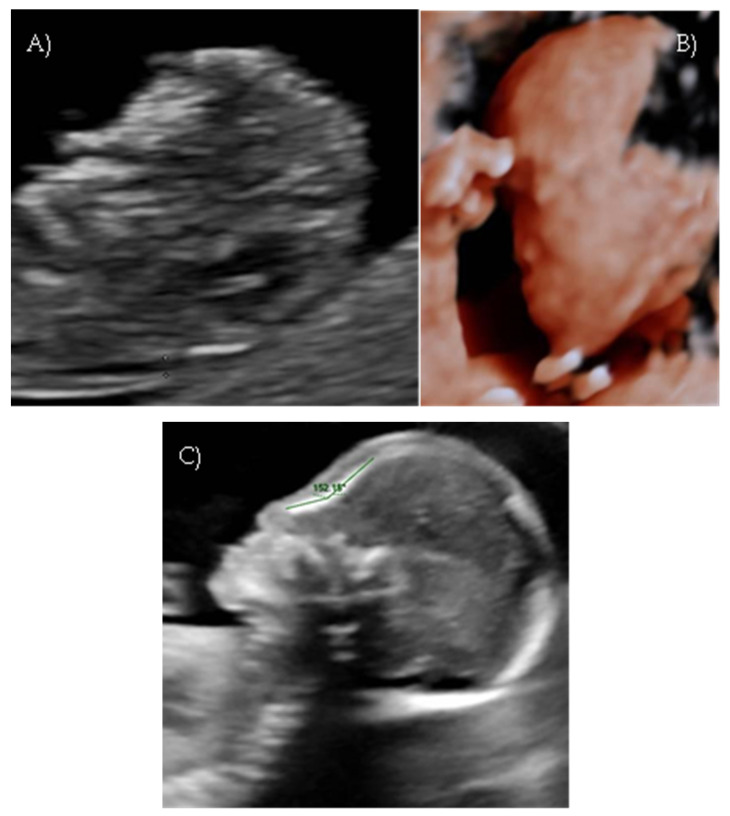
Case 1*. (A) First-trimester 2D ultrasound Fetal profile. (B) Flat fetal profile, 21 weeks and 1 day, 3D reconstruction. (C) Nasal-frontal angle: 152.18°.
